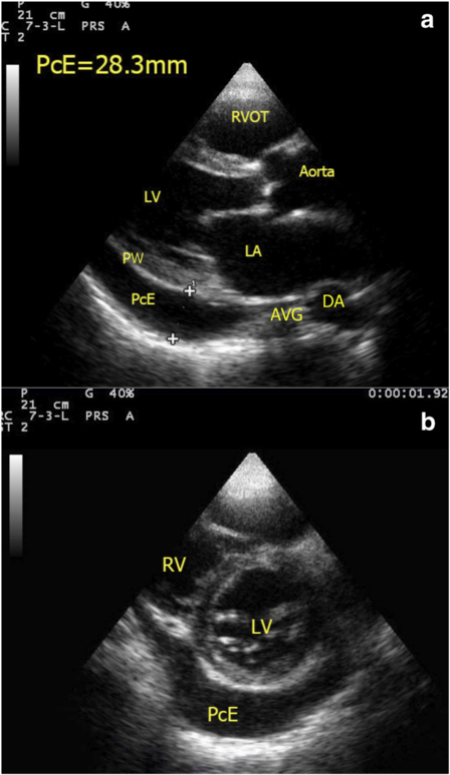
a, b Pericardial effusion (*PcE*). A large amount of pericardial effusion (*PcE*) is seen in both parasternal long axis and short axis view. The maximum size of the pericardial effusion measures 2.83 cm. Note the relationship between the pericardial effusion and the descending aorta (*DA*). (*RV* right ventricle, *LV* left ventricle, *PW* posterior wall of LV, *PcE* pericardial effusion, *LA* left atrium, *RVOT* right ventricular outflow tract, *DA* descending aorta, *AVG* atrioventricular groove)
